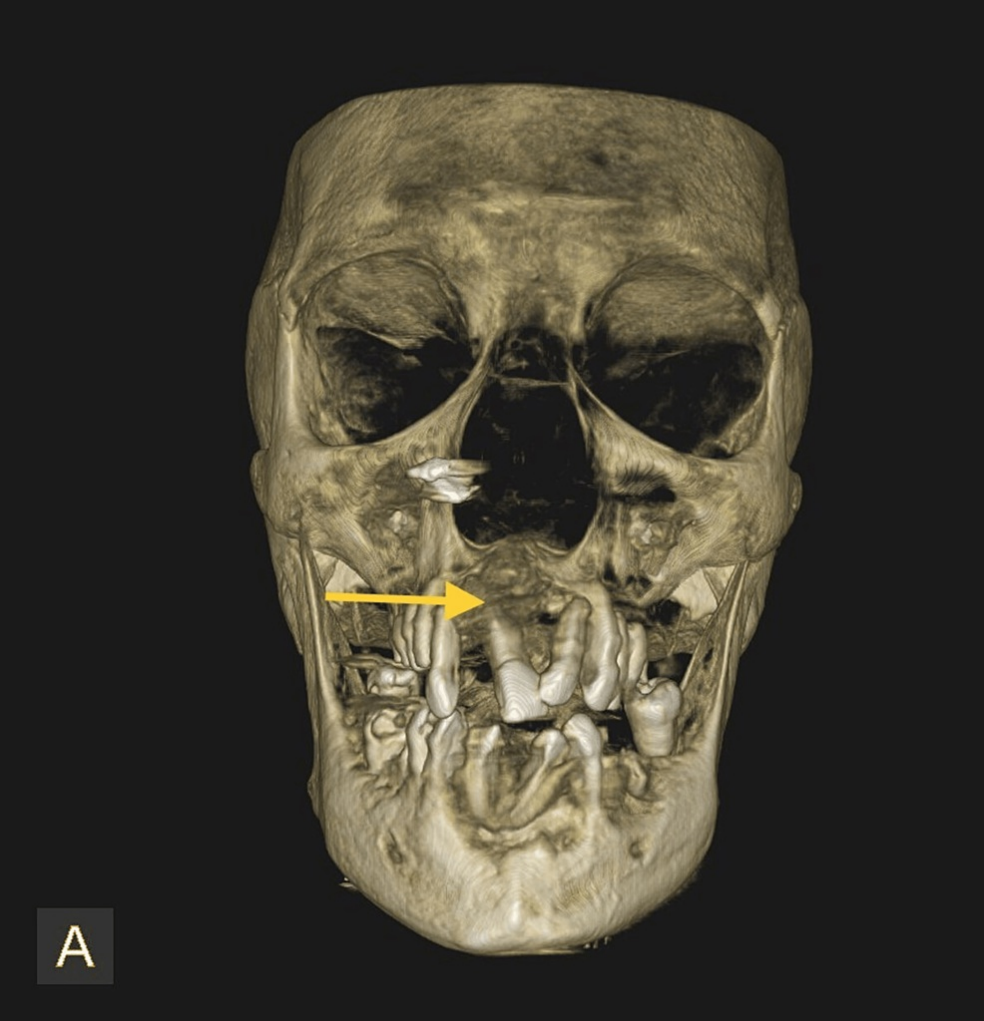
Pre-operative cone beam computed tomography (CBCT) Represents the 3D CBCT showing increased thickness of frontal bone, parietal bone, and maxilla. Obliteration of marrow spaces, alteration of trabecular pattern showing cotton wool/ground glass appearance. The arrow mark represents the displacement of teeth in the anterior maxilla.
